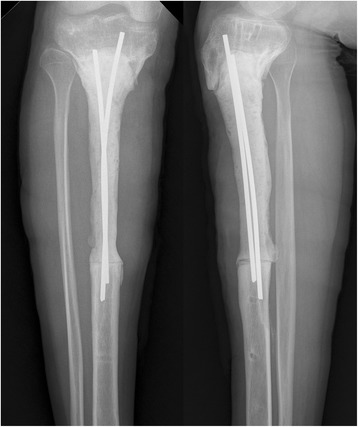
Anteroposterior and lateral radiographs of right tibia after removal of the intercalary allograft and reconstruction with temporary cemented spacer
